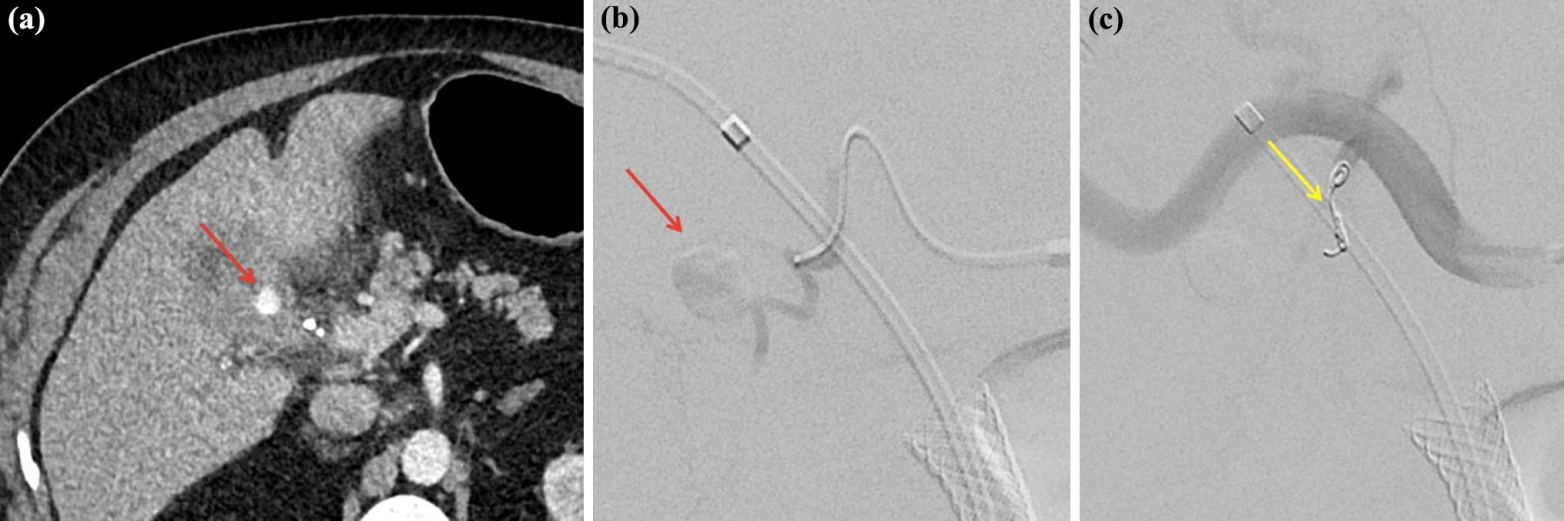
Arterial phase of triple phase contrast enhanced computed tomography in axial plane demonstrating arterial hyperenhancement (red arrow) a. Cystic artery pseudoaneurysm (red arrow) confirmed on angiography b with subsequent deployment of coil (yellow arrow) with successful embolization c
